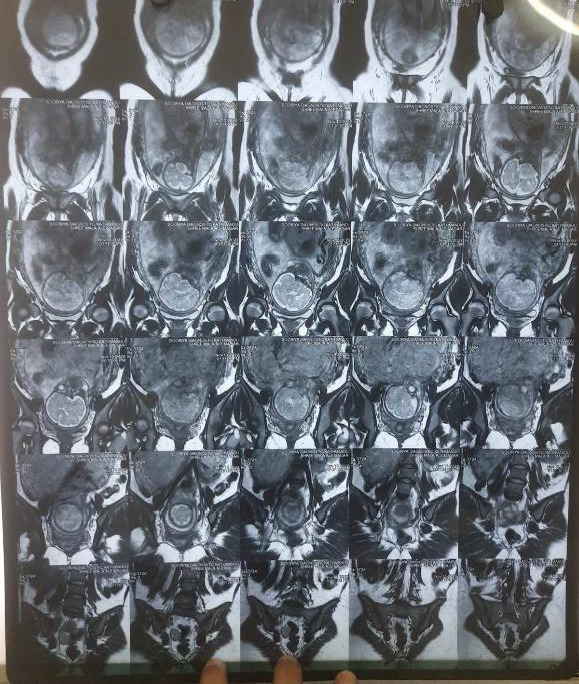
MRI picture of pregnancy with metastatic mass in the pouch of Douglas.
